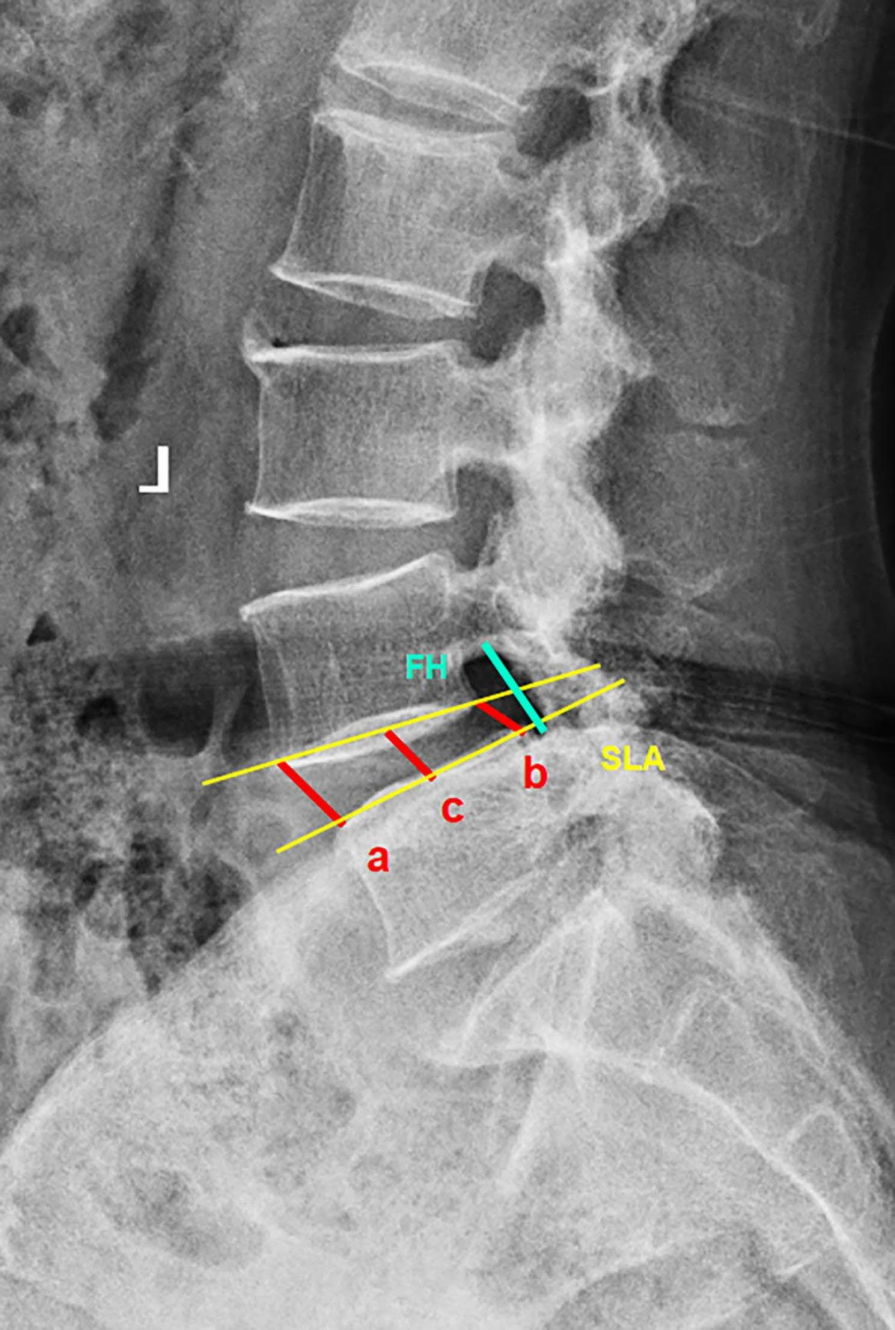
Measurements of radiographic parameters. disc height (a: ADH, anterior disc height, b: PDH, posterior disc height, c: MDH, middle disc height), segment lordosis angle (SLA), and foraminal height (FH).
